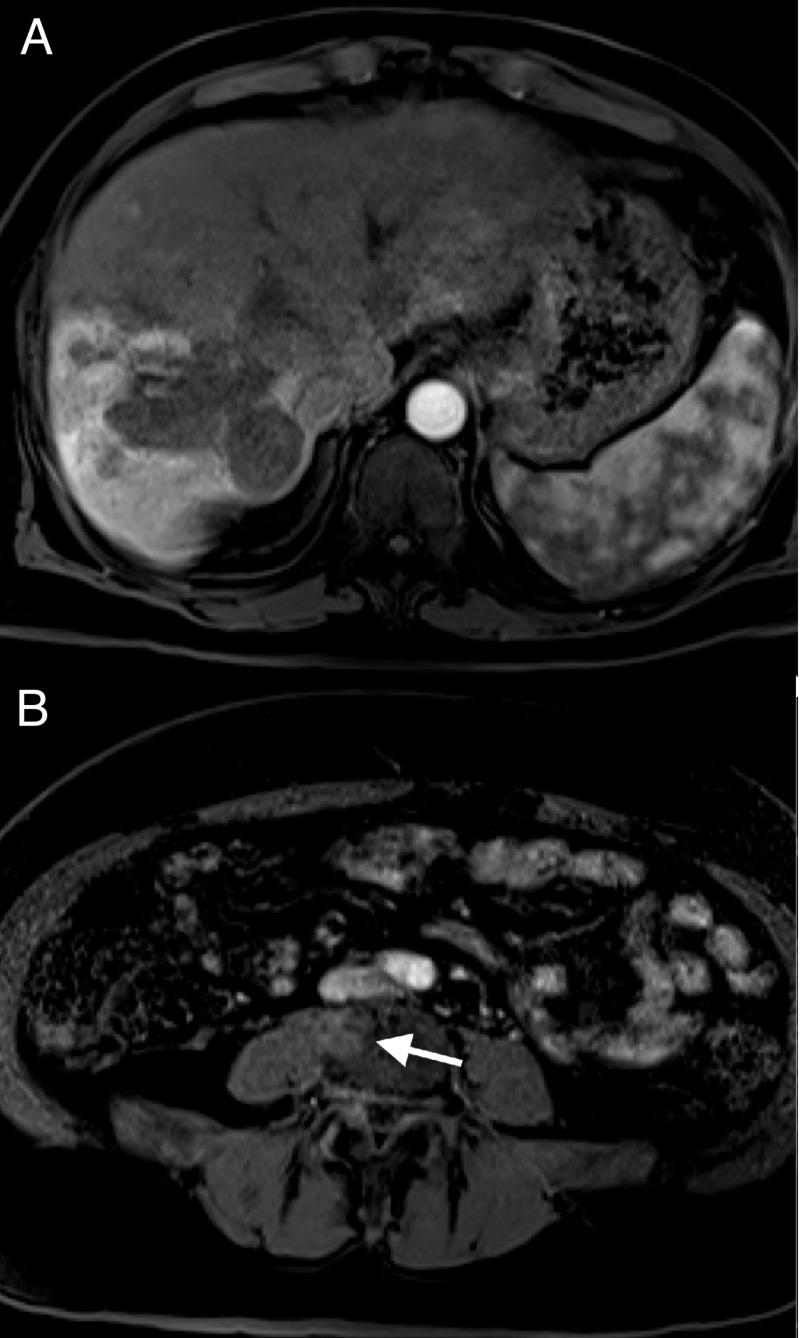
December 2017 MRI with IV contrast arterial phase 20 second (T1) vibe sequence (A) Contraction of the right hepatic lobe with perfusional changes from Y-90, decreased right hepatic lobe tumor size, and markedly reduced enhancement; (B) decreased enhancement of the L4 lesion MRI: magnetic resonance imaging, IV: intravenous
